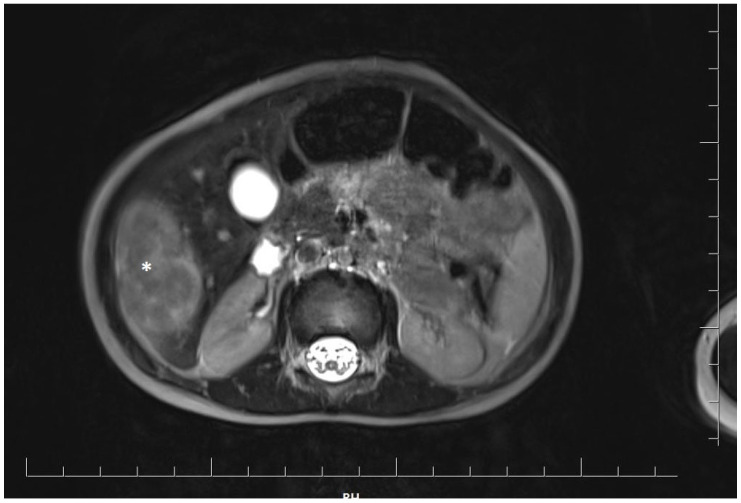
Magnetic resonance imaging of the abdominal cavity showing well-defined, polycyclic, heterogeneous lesions in the right hepatic lobe. The tumor is marked with an asterisk.
